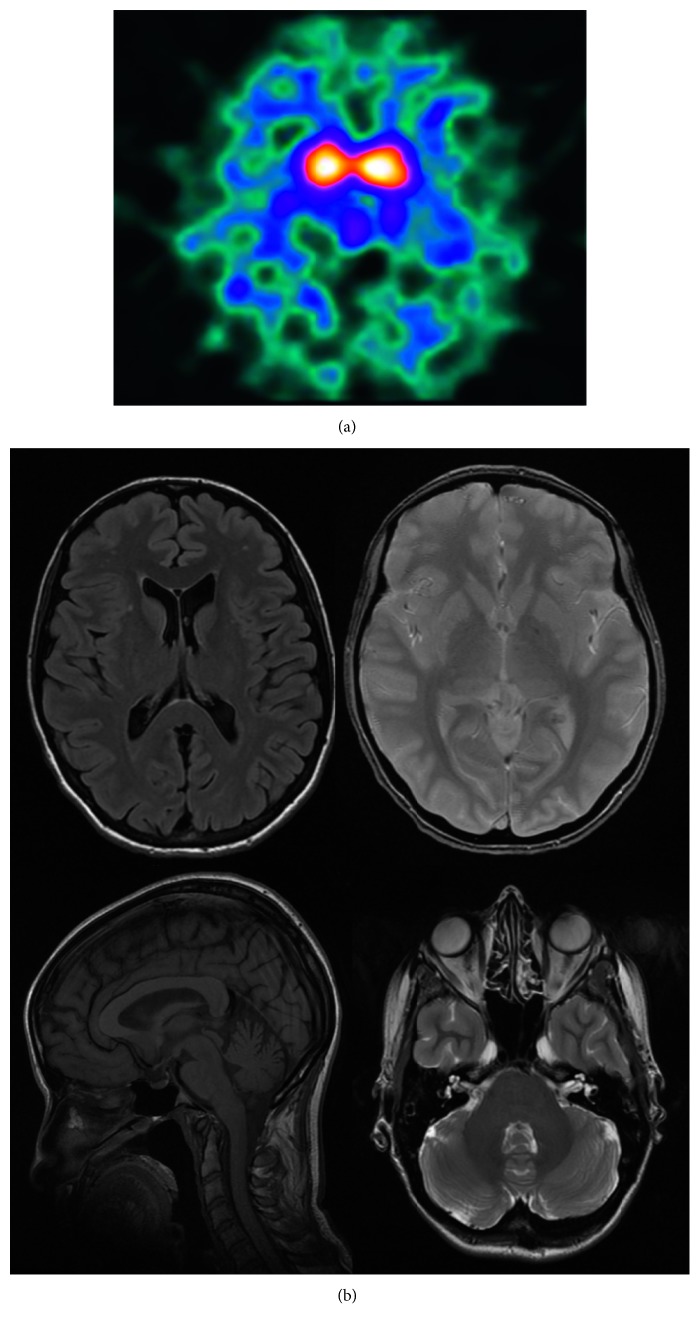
DaTSCAN and magnetic resonance imaging. (a) DaTSCAN image showing a reduced tracer uptake in both striatal regions, especially on the right side. (b) Magnetic resonance imaging (MRI) scan showed a normal profile with aspecific gliotic microvascular outbreaks in subcortical white matter of front-insular hemispheres. Axial Flair MRI scan shows aspecific gliotic microvascular outbreaks in subcortical white matter of front-insular hemispheres; Axial GRE MRI scan shows normal putamina bilaterally in absence of degeneration signs or deposits; sagittal-T1 MRI scan shows normal midbrain; axial-T2 MRI scan shows normal cerebellum.
